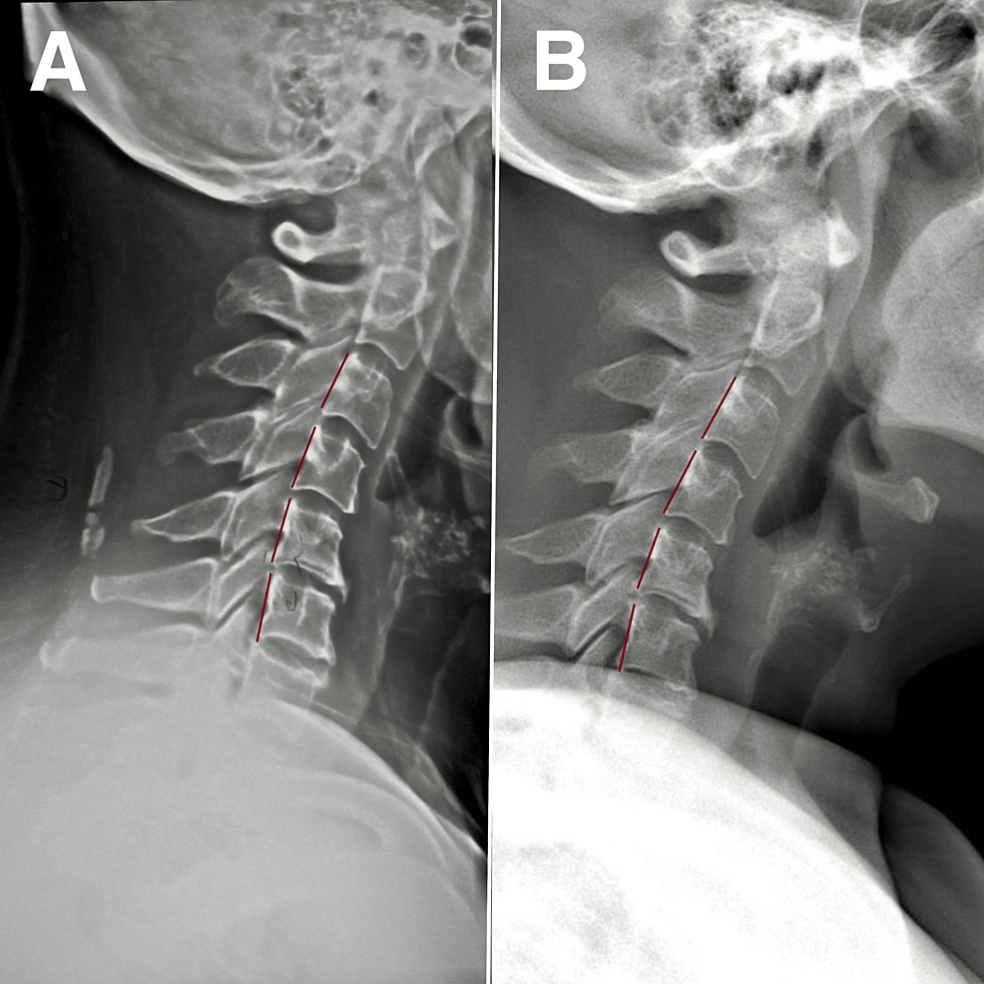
Cervical radiographs (A) Lateral cervical radiograph taken in 2017 showed moderate spondylosis and reversed cervical lordosis. (B) Lateral cervical radiograph taken in 2020 showed worsening of cervical lordosis (as indicated by the red lines).
